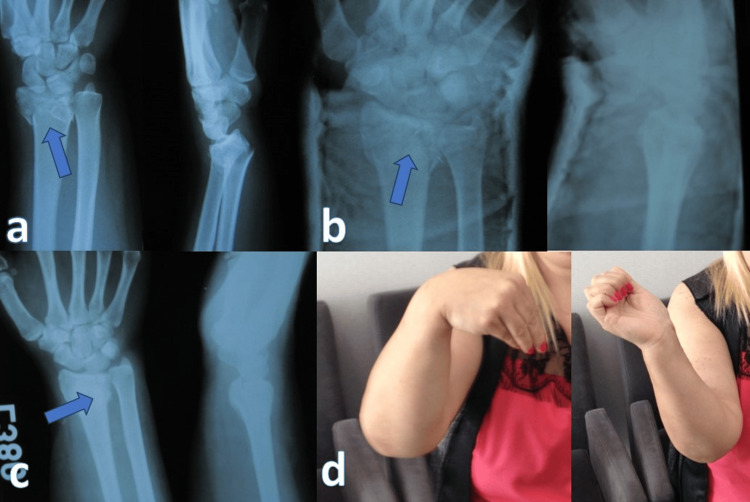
Conservative treatment. (a) Intra-articular comminuted distal radius fracture before reduction and intra-articular fragment (arrows). (b) Radiographic image of the distal radius in a cast after closed reduction. (c) Third-month control radiograph and first-year clinical image. (d) Good clinical outcome despite some dorsal angulation at the end of the follow-up.
